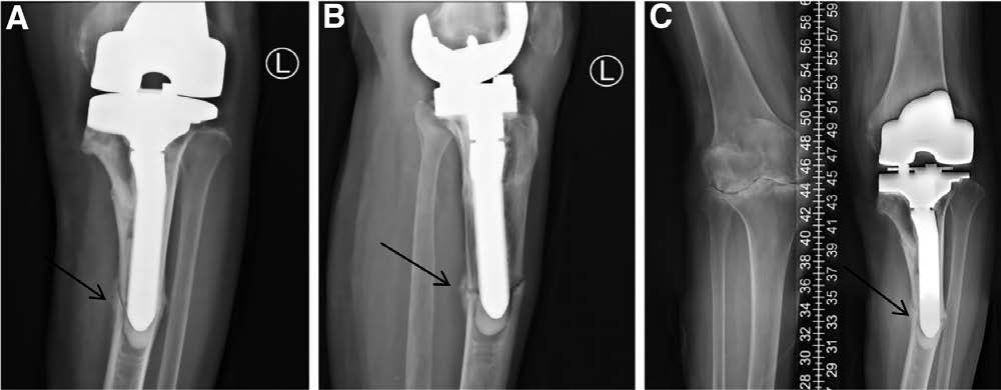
X-ray diagnosis of the tibia fracture of the middle and upper parts, slightly more distal to the original preoperative stress fracture, without any trauma. (→ represents the PPF in the left knee joint).
